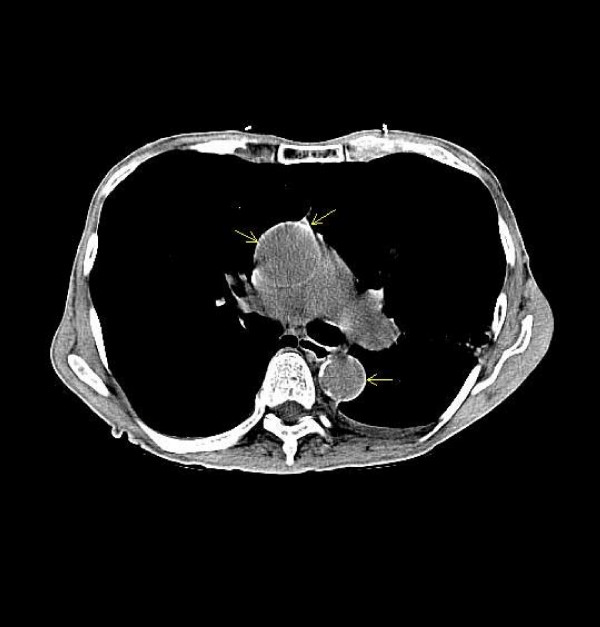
Computed tomography of the chest showing extensive calcification of ascending and descending aorta. This classically looks like a 'solar eclipse'.
